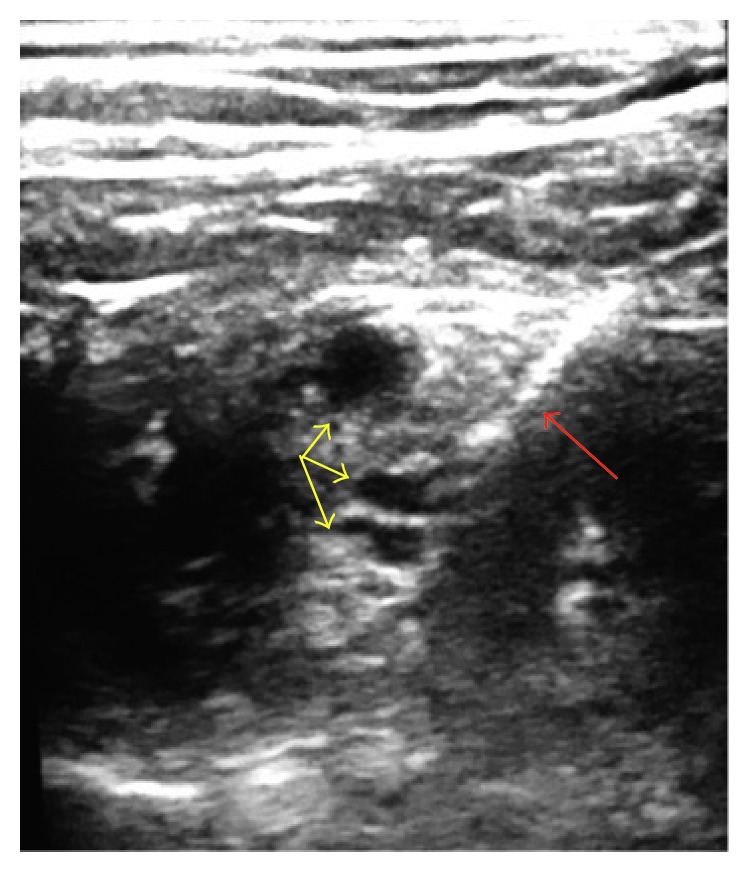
Chemical neurolysis of the right brachial plexus under ultrasound guidance. Red arrow: echogenic needle. Yellow arrows: brachial plexus at the level of the trunks.
